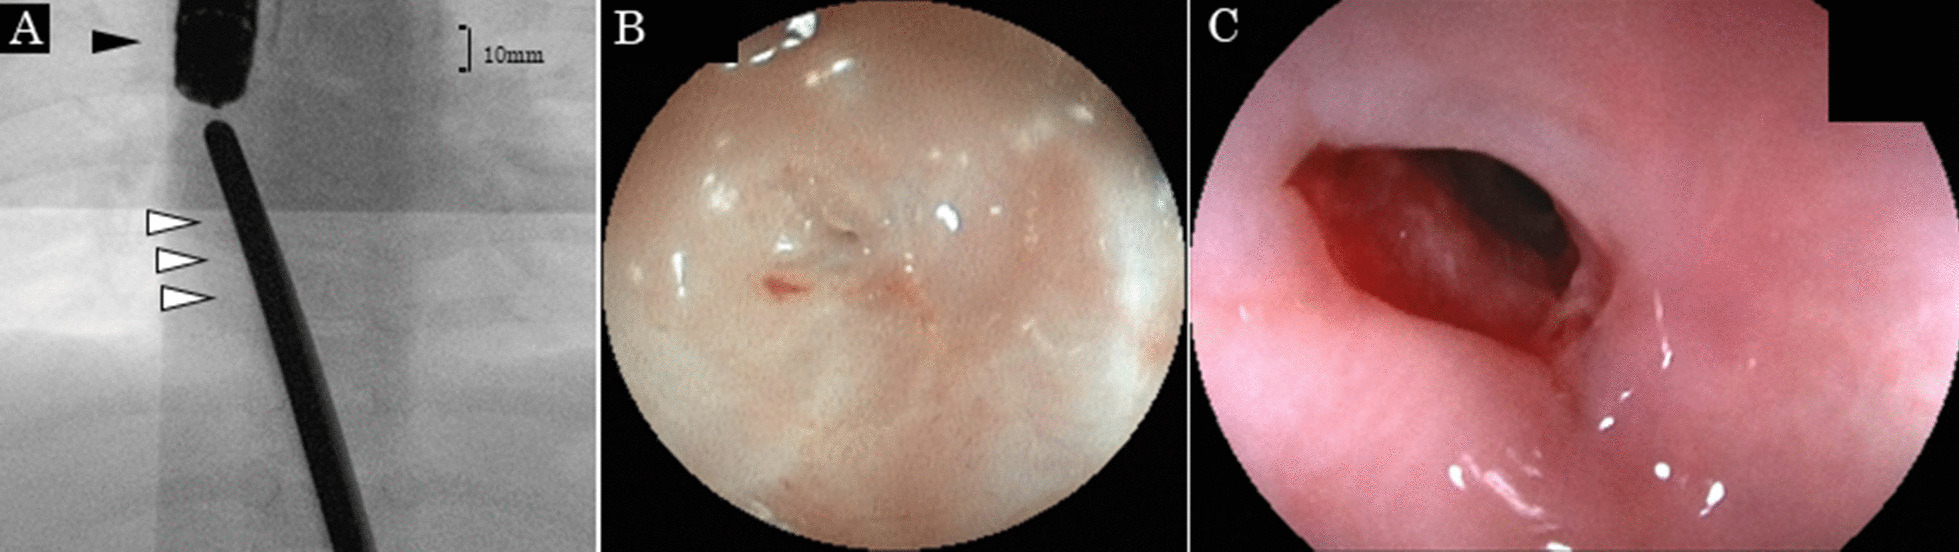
Fluoroscopic and endoscopic findings after puncture and endoscopic balloon dilation. A Pean forceps were inserted via the gastrostomy toward the caudal side of the anastomotic site. Contrast radiography showed that the length of the stenosis was several millimeters (black arrow, peroral conventional endoscope; white arrow, Pean forceps via gastrostomy). B An ultrathin endoscope was inserted via the gastrostomy after the puncture. The puncture hole was present at the center of the lumen. C After endoscopic balloon dilatation had been performed three times, the hole was deemed sufficiently dilated
